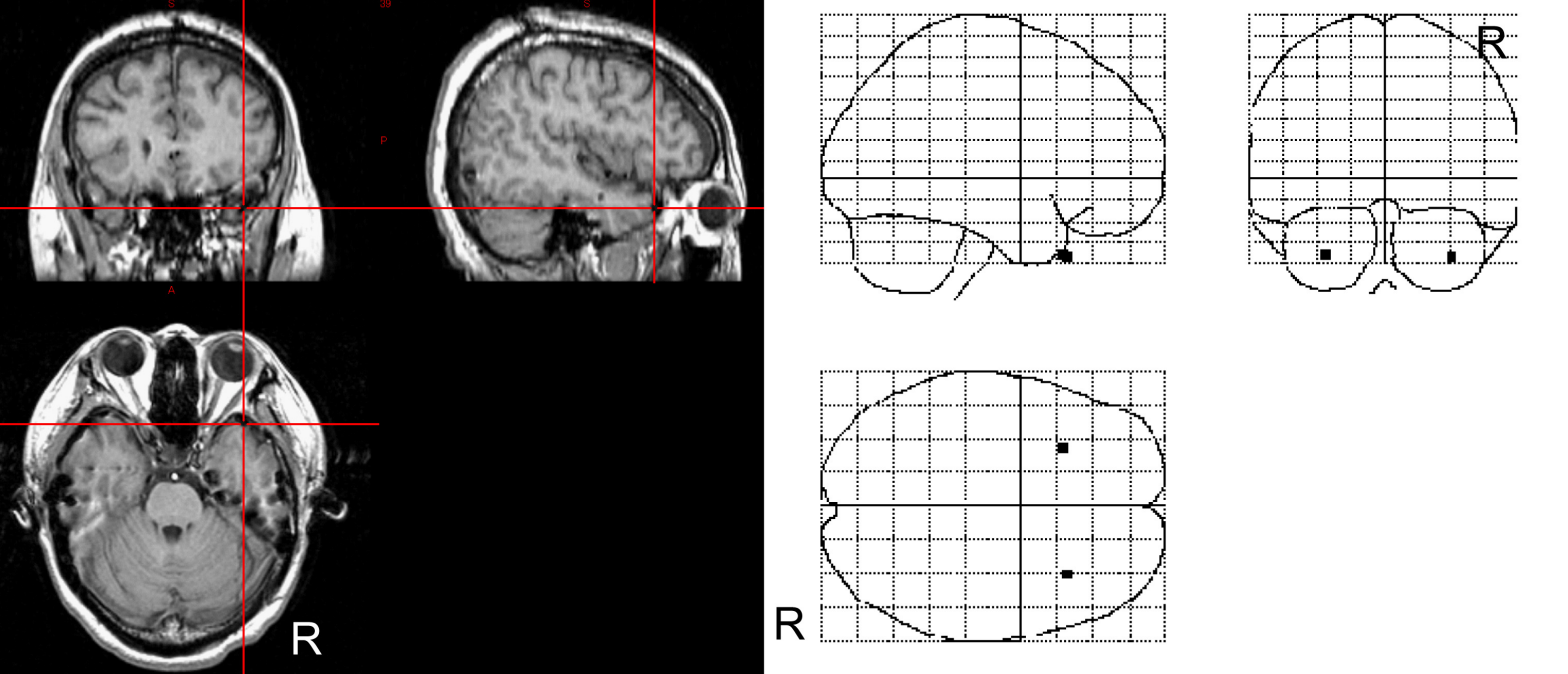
Location of electrodes in the temporal pole. Left) Representative anatomical magnetic resonance images. Cross hairs indicate the electrode location in the temporal pole. Right) Averaged coordinates of the electrodes in the temporal pole in the Montreal Neurological Institute space.
